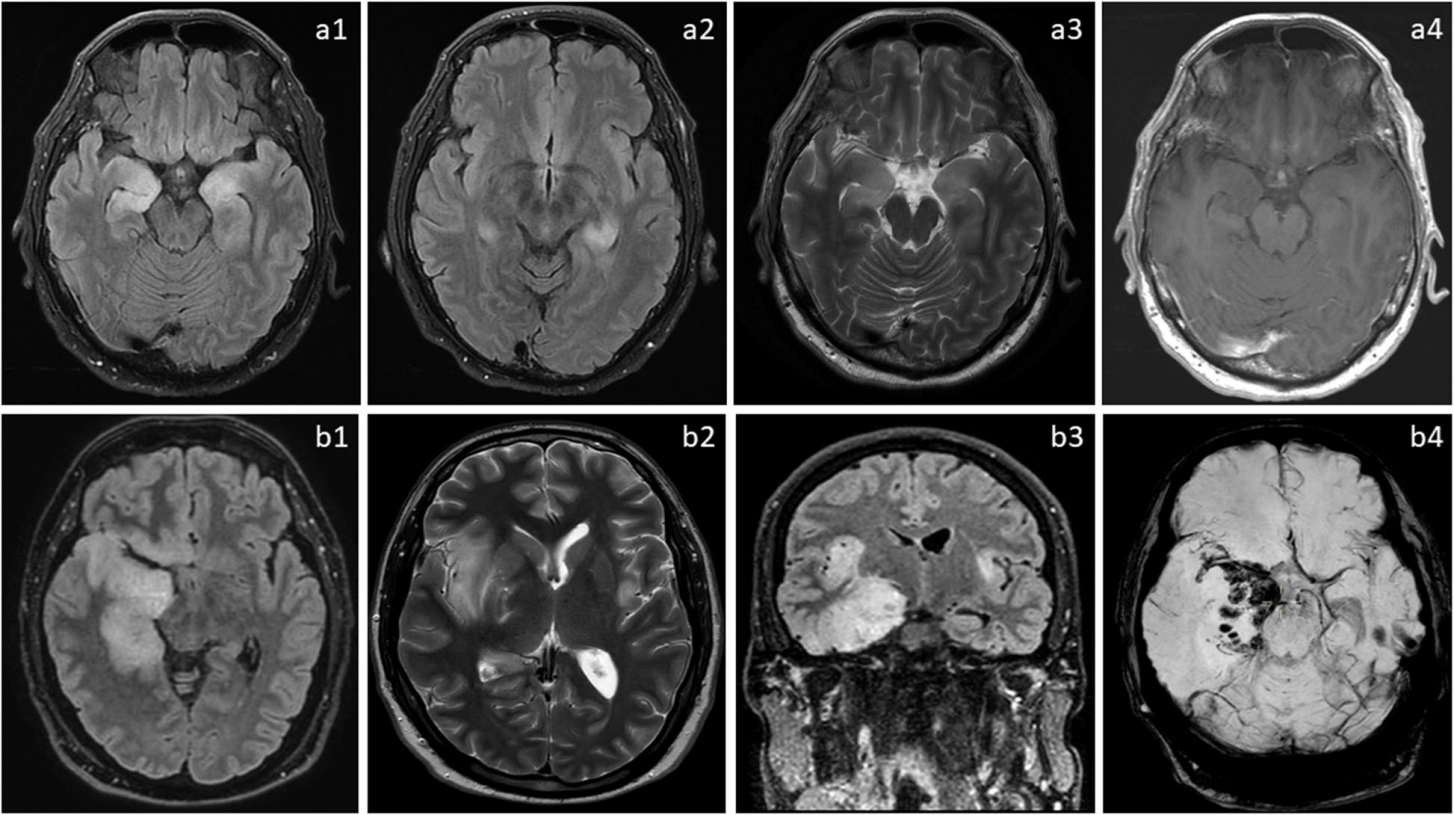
MRI characteristics of limbic encephalitis. a A 53-year-old male, presenting with headache and drowsiness, tested positive for Leucine-rich, glioma inactivated 1 (LGI1) antibody. MRI depicted symmetrical FLAIR (a1, a2) and T2 (a3) signal abnormalities in bilateral medial temporal lobes. No enhancement was observed in the T1 post-contrast sequence (a4). b Comparative case: highlighting a case of herpes encephalitis, a primary differential for autoimmune limbic encephalitis. A 36-year-old man diagnosed with herpes simplex encephalitis displayed involvement of the right medial temporal lobes and bilateral insular cortices, more severe on the right side, showcasing elevated FLAIR (axial b1 and coronal b3) and T2 (b2) signal intensities. Blooming artifacts in the right medial temporal lobe on SWI sequence (b4) suggested hemorrhage, favoring herpes simplex encephalitis over autoimmune encephalitis
